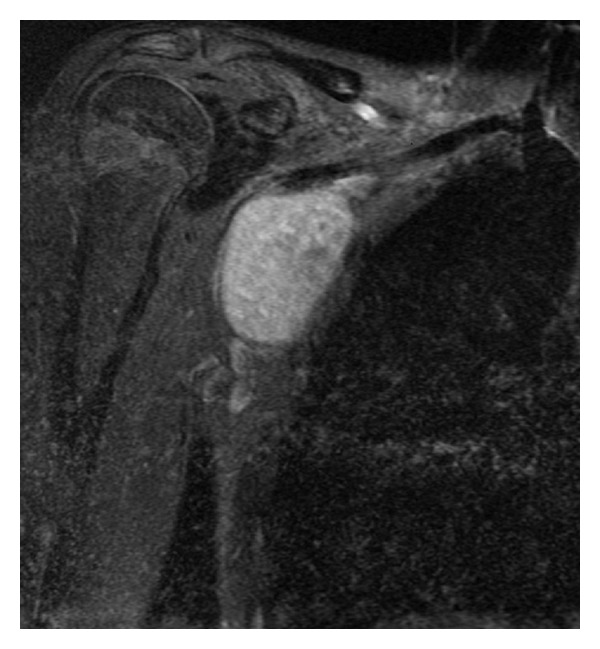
Following intravenous contrast administration, there is homogeneous enhancement throughout the lesion on a fat-suppressed T1-weighted (450/22) coronal image.
